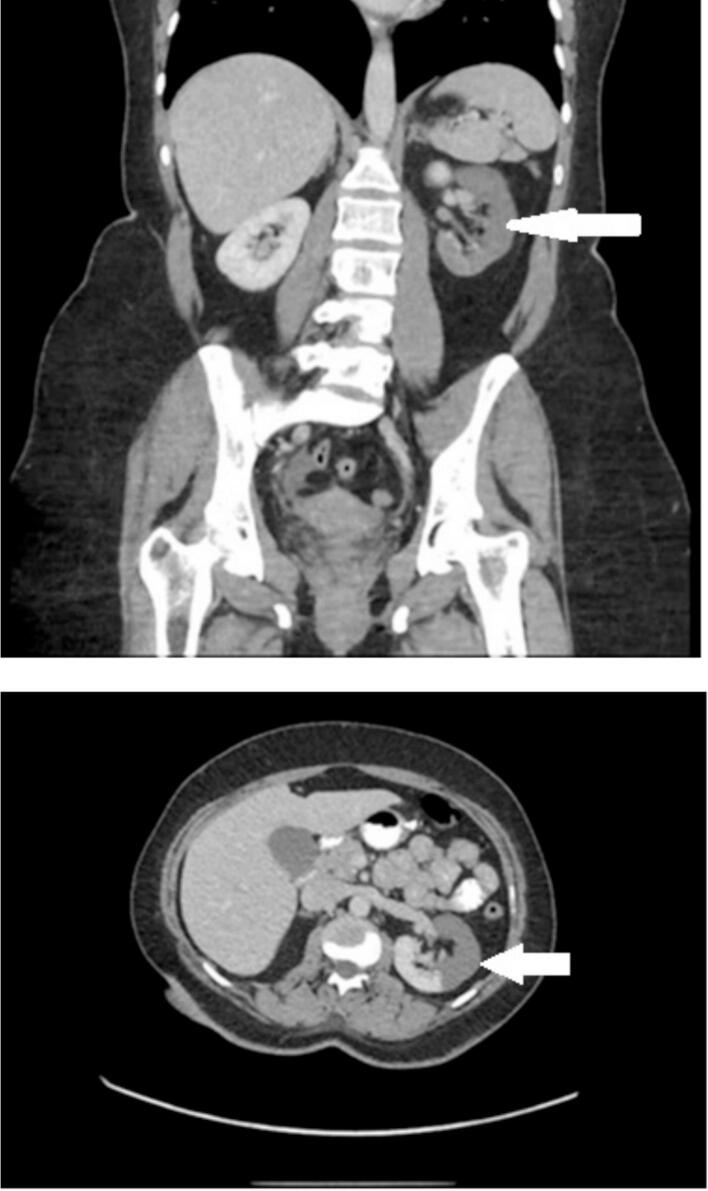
An enhanced CT scan of the patient showing hypoperfusion of the left kidney, affecting approximately 60–70 % of the organ with sparing of the posterior medial region. Renal arteries and vein were patent.
